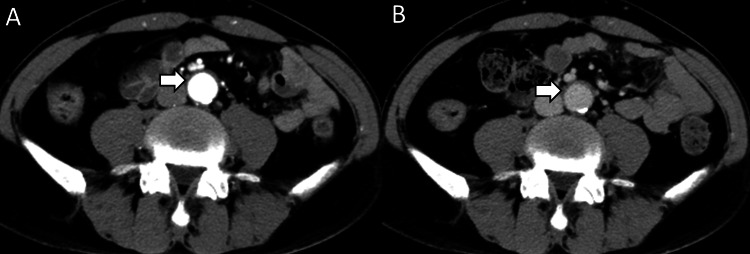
Contrast-enhanced computed tomography findings showing post-treatment periaortitis. (A) Arterial and (B) equilibrium phase of contrast-enhanced computed tomography five months after the start of treatment: the abdominal aortic wall thickening had disappeared (arrow).
